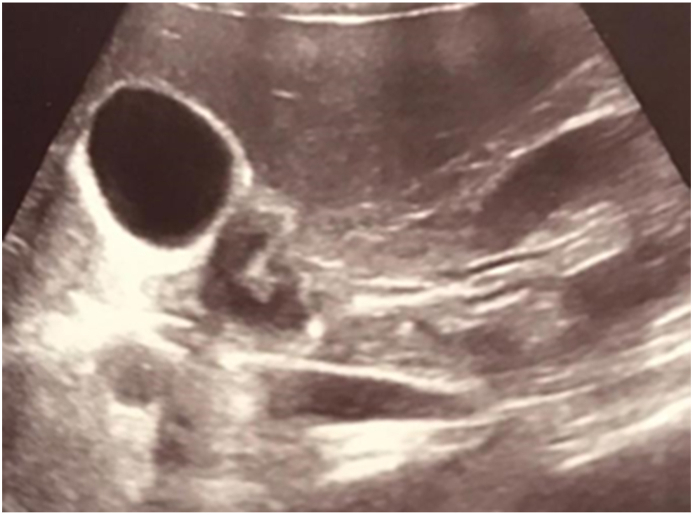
Abdominal Ultrasound showing Focal area of Wall thickening involving the first part of the duodenum.
